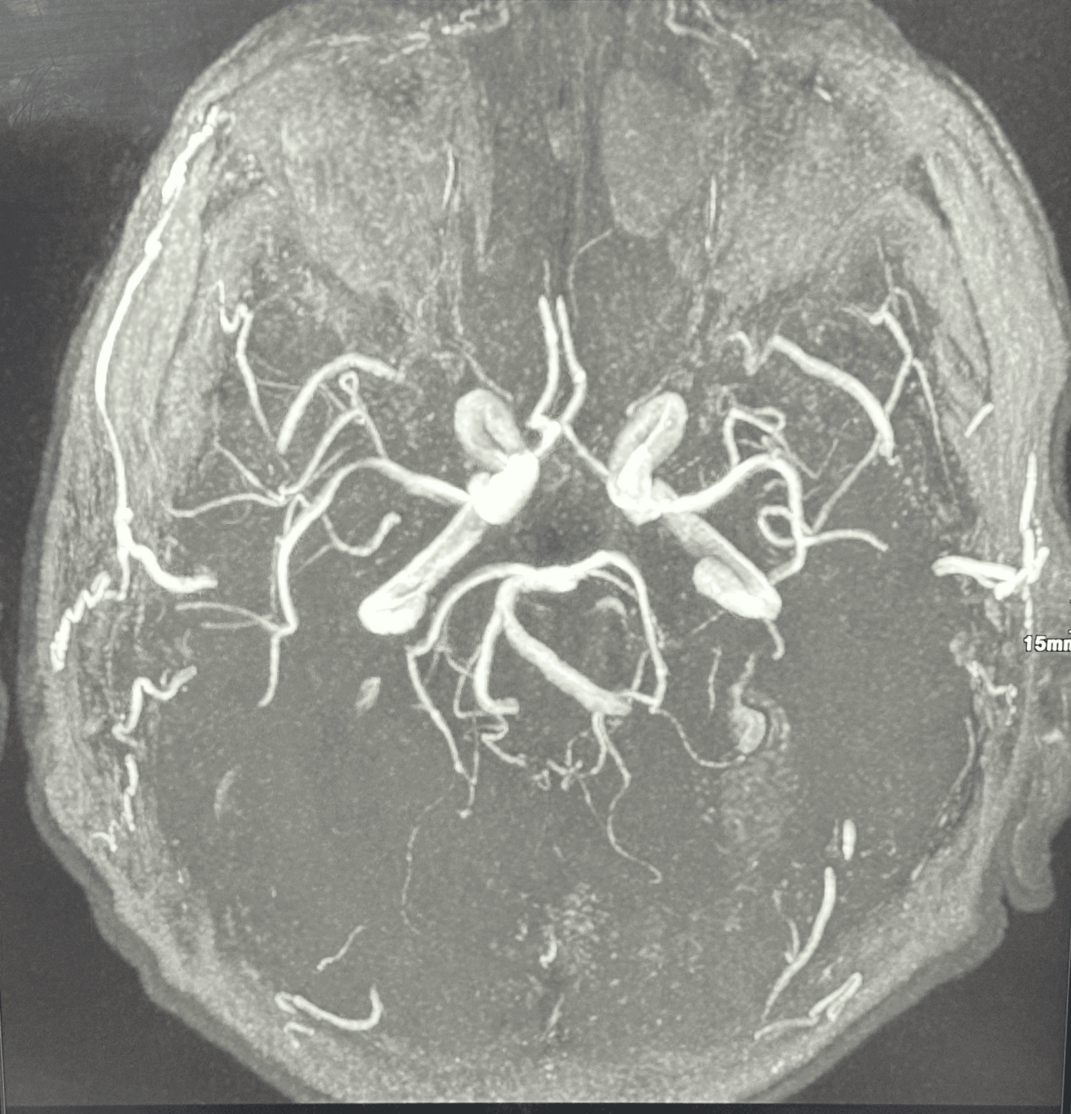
Preoperative cerebral MRI angiography Normal caliber and patency of the intracranial vessels with no significant stenosis or aneurysm. MRI: magnetic resonance imaging
